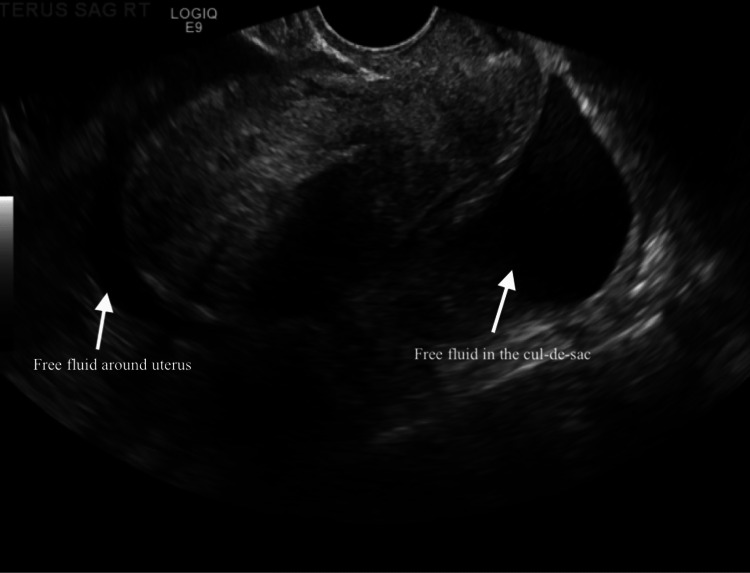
Ultrasound showing hemorrhaging around the uterus and cul-de-sac.
